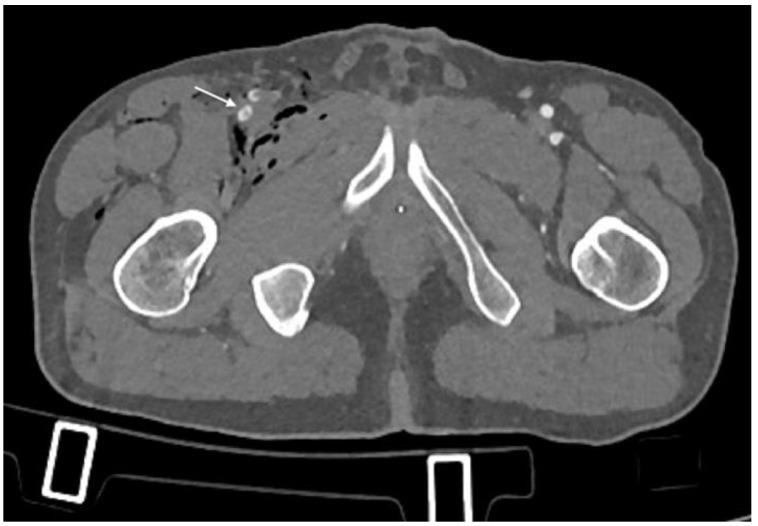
CTA, axial planes. Right deep femoral artery dissection can be seen (arrow), resulting in a linear flap within the vessel lumen.
